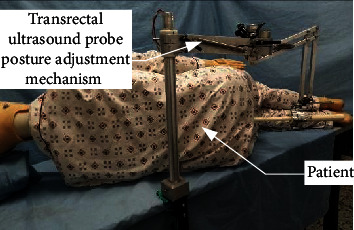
Physical prototype of the posture adjustment mechanism of ultrasonic probe.
